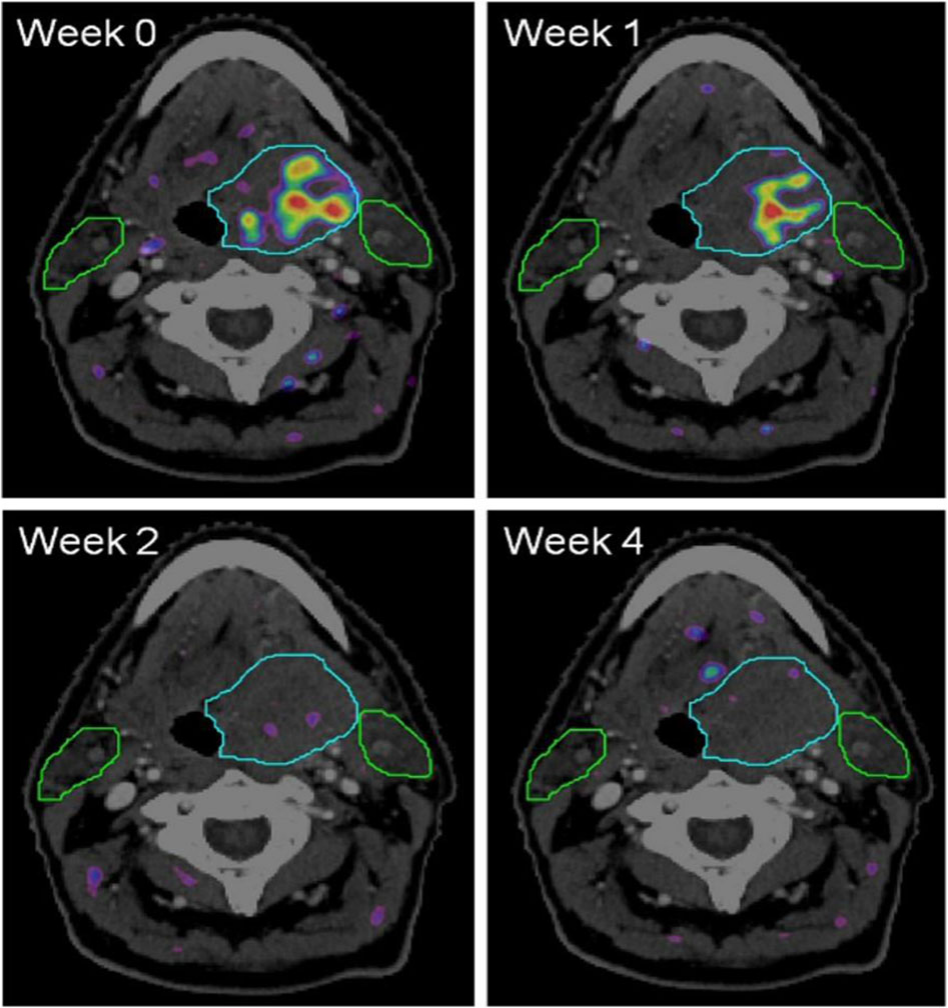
^18^F-FAZA, therapy evaluation of a brain lesion. *PET/CT findings*: four FAZA-PET-CT scans made at different weeks prior (week 0) and during (week 1, 2, and 4) the course of chemoradiation. The rainbow colours depict the amount of FAZA uptake. The light blue line depicts the extent of the primary tumour situated in the base of tongue. The green lines depict the extent of the parotid glands (left and right). Note that in week 2 and 4 no increased FAZA uptake is visible any more, demonstrating that the hypoxic area in week 0 and 1 disappeared
